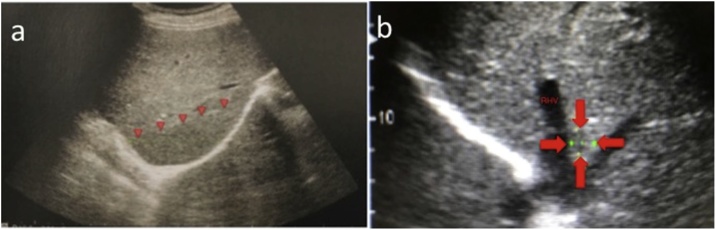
Abdominal ultrasonography showing (a) a hypoechoic lesion measuring 61 mm in diameter located in the posterior section and (b) a hypoechoic lesion measuring 10 mm in diameter at the root of the right hepatic vein.
